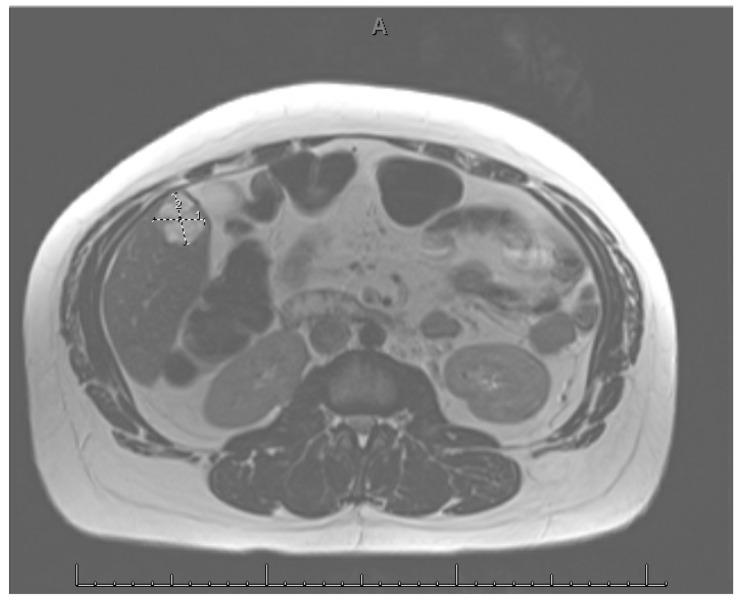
Abdominal MRI. Right Hepatic Lobe Mass.
